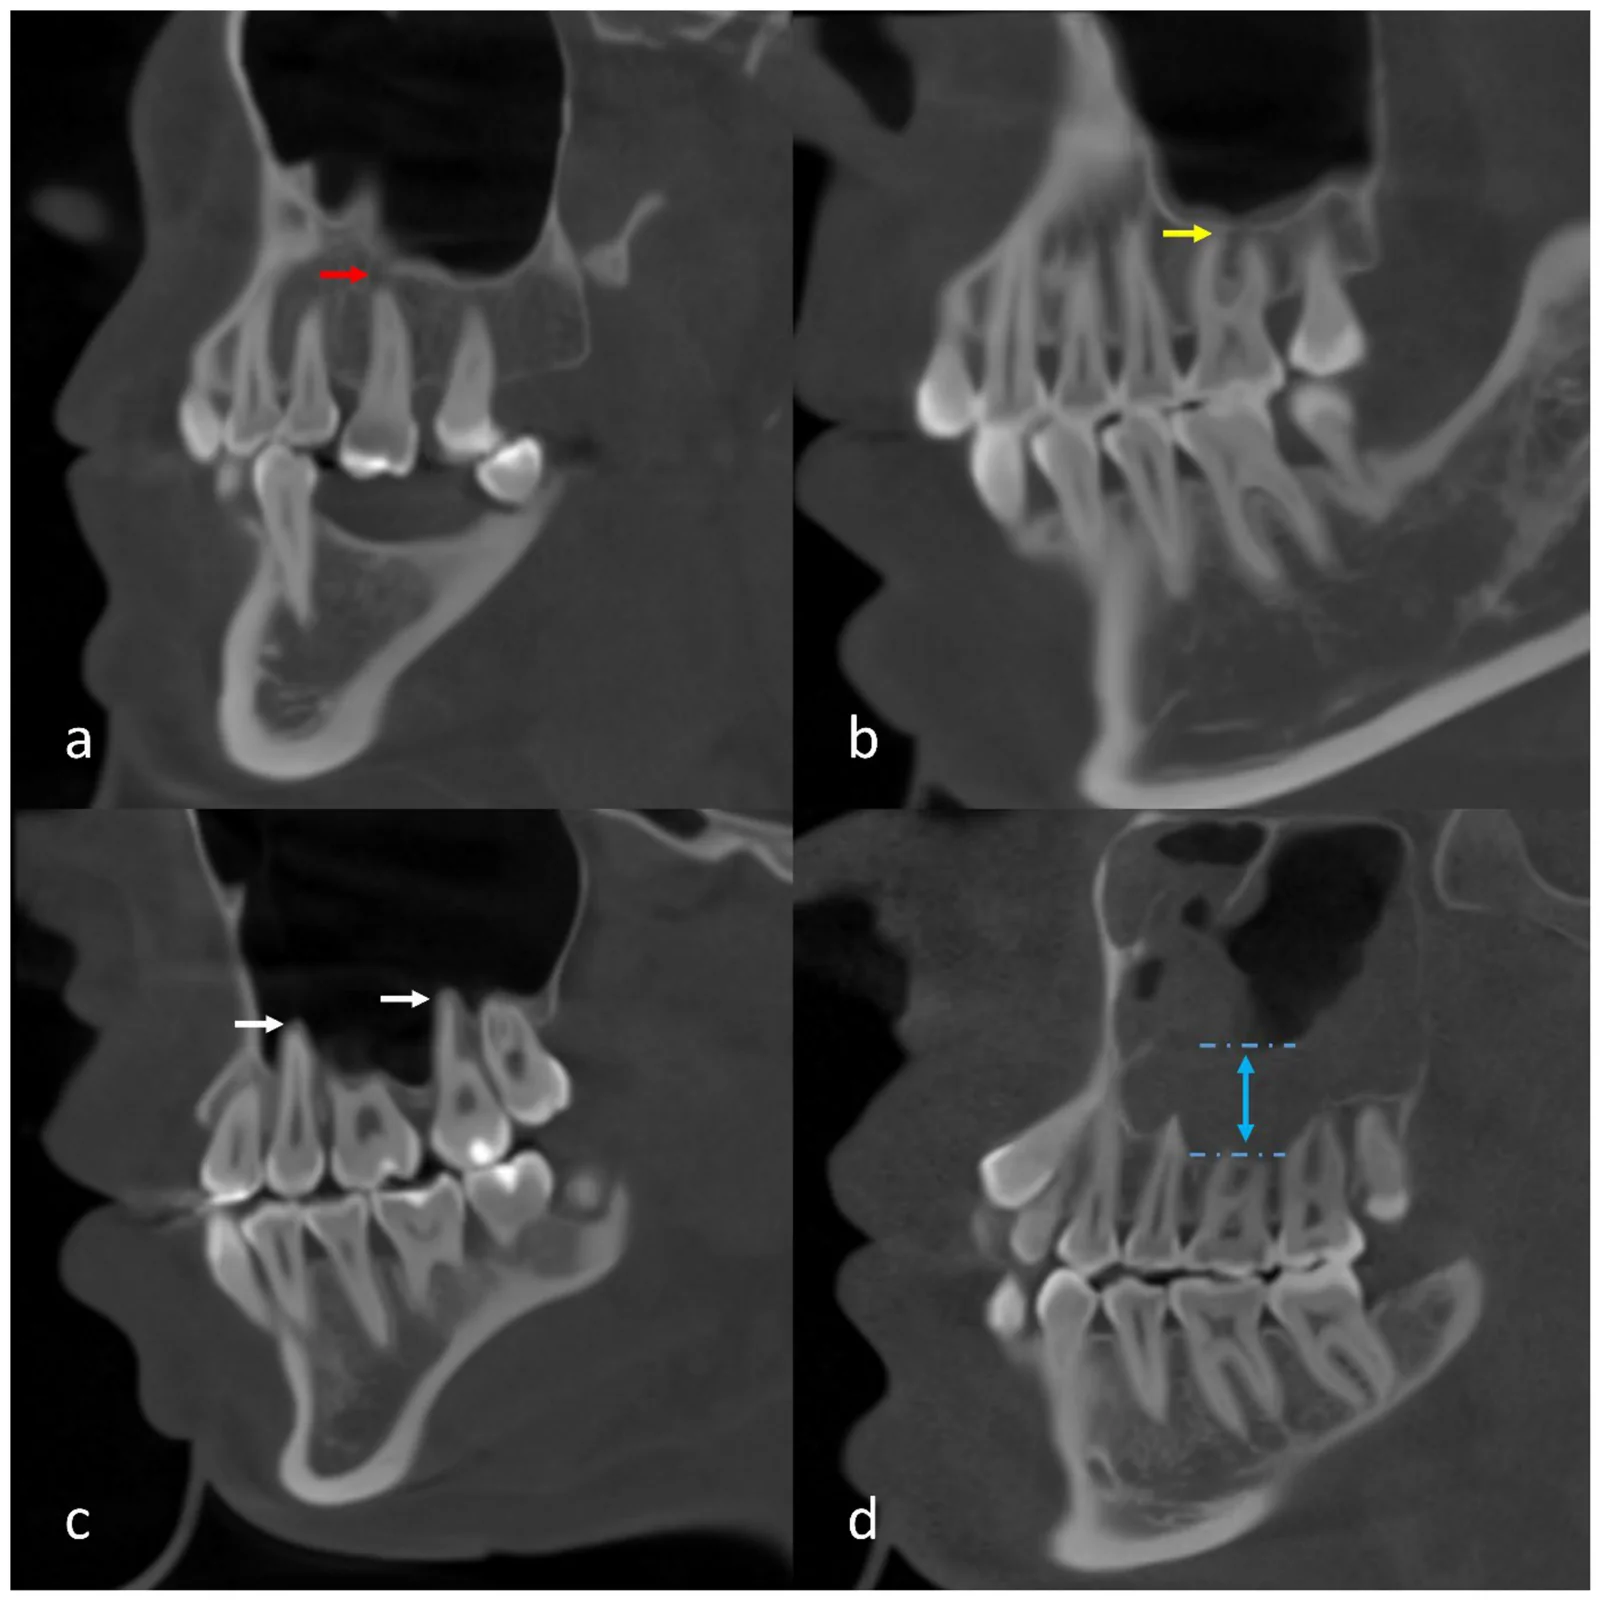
Representative CBCT images demonstrating the classification of anatomical proximity between maxillary posterior tooth roots and the sinus floor, along with mucosal thickening (MT) measurements. (a) Type 1: The root apex (red arrow) is located below the maxillary sinus floor without contact. (b) Type 2: The root apex is in direct contact with the cortical boundary of the sinus floor (yellow arrow). (c) Type 3: The root apices protrude into the maxillary sinus cavity (white arrows). (d) Severe mucosal thickening in a Type 3 relationship: mucosal thickening exceeding 10 mm, with the blue double-headed arrow indicating the measurement.
